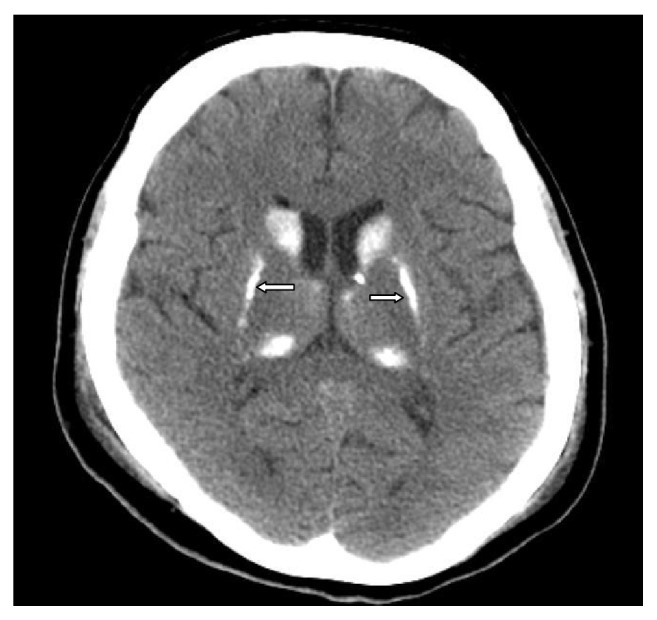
Bilateral symmetrical calcifications are seen in the basal ganglia of the brain CT.
